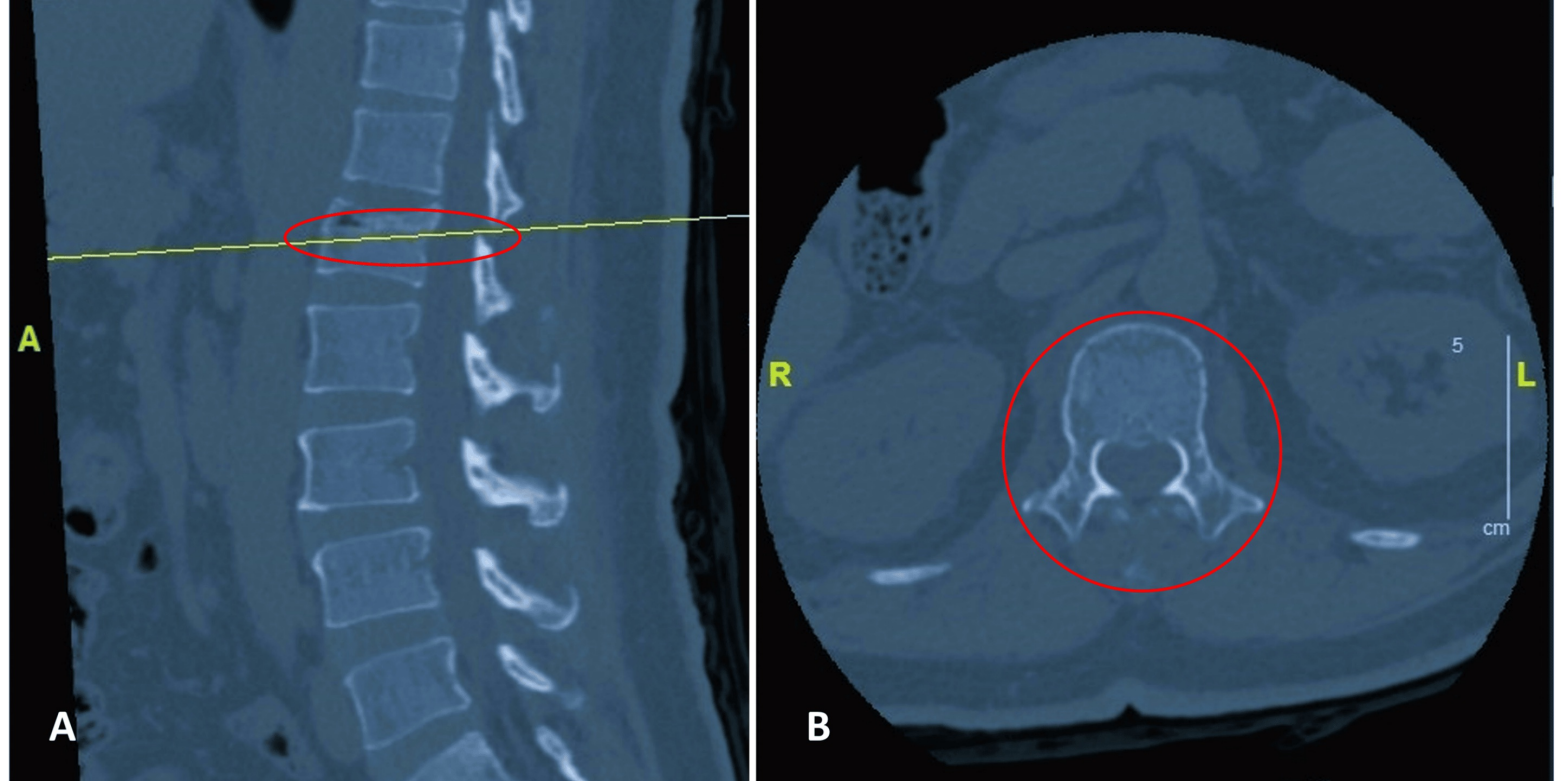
A: Sagittal view indicates the spinal cord level where the CT slice was taken. B: CT scan of the lumbar spine shows an L1 burst fracture classified as A3 according to the comprehensive classification system for spinal injuries CT: computed tomography
